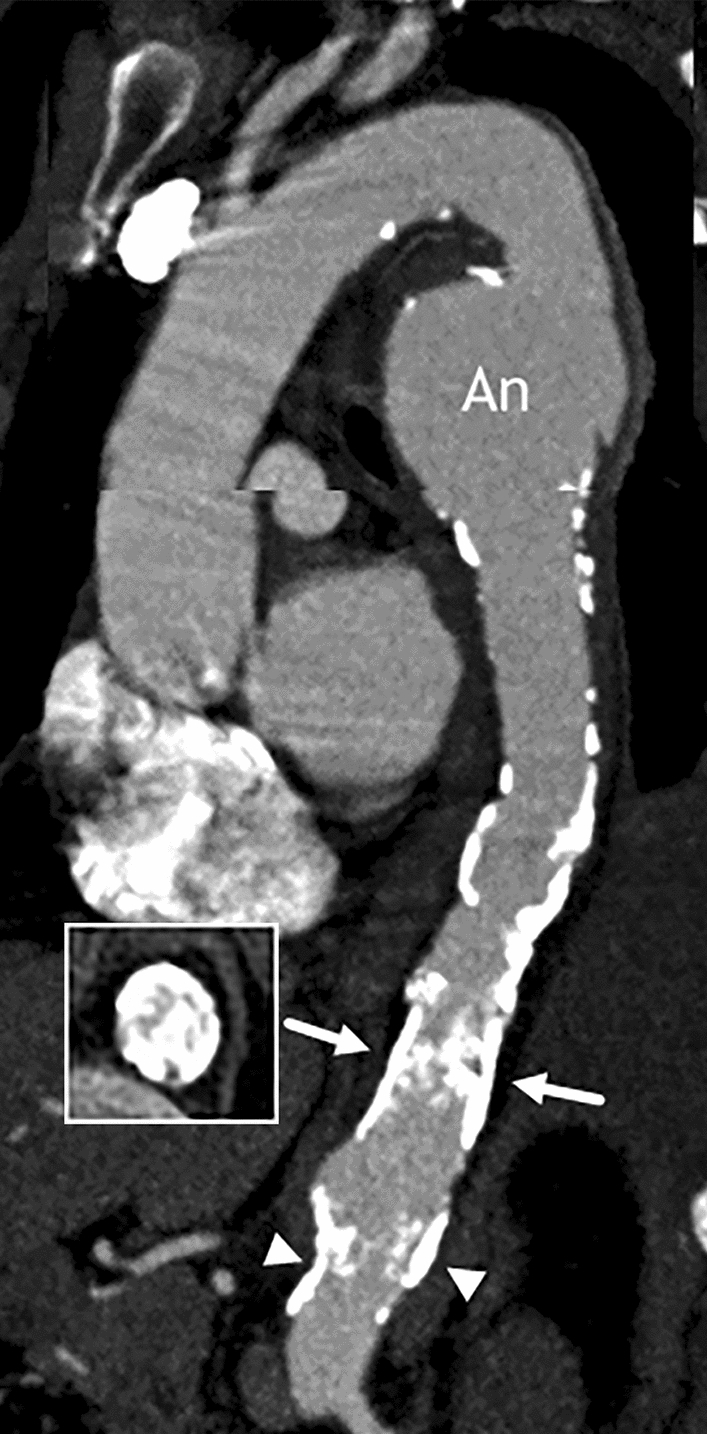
Pre-interventional CTA (composed reformatted images): Five-cm-wide false aneurysm of the proximal descending thoracic aorta (An). Obstructive wall calcifications at the aortic hiatus (arrows). Nonobstructive calcifications in the suprarenal abdominal aorta (arrowheads)
